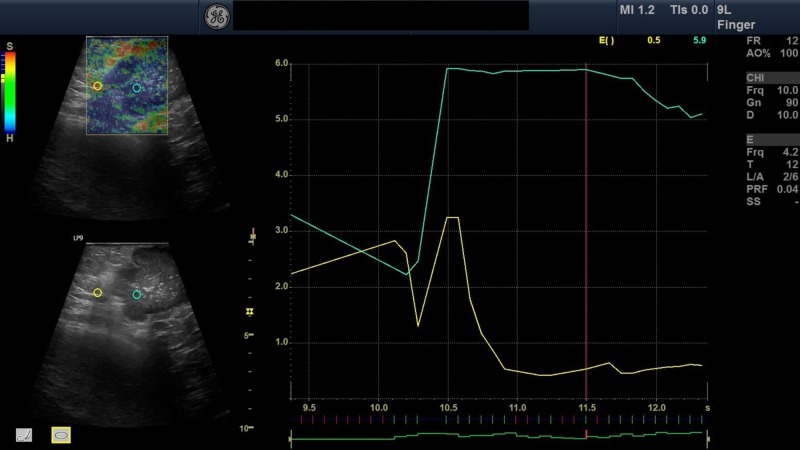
Elastography image of breast lesion A 45-year-old woman presented with a concern of right side breast mass with pain and discharge from the nipple. On palpation, the mass was hard. The mass was in the upper outer quadrant involving two-thirds of the breast parenchyma. B-mode ultrasonography of breast was done followed by strain elastography. On ultrasonography, a large ill-defined lobulated heteroechoic mass was seen with few foci of calcification and increased vascularity. The mass was graded as BI-RADS grade 4C. On elastography, two regions of interests were taken represented by green and yellow circles. The yellow and green region of interest represents normal area and area of mass respectively with their graphic representation (left side). Elastography score of the lesion was 4 and strain ratio of the lesion was 3.9.
